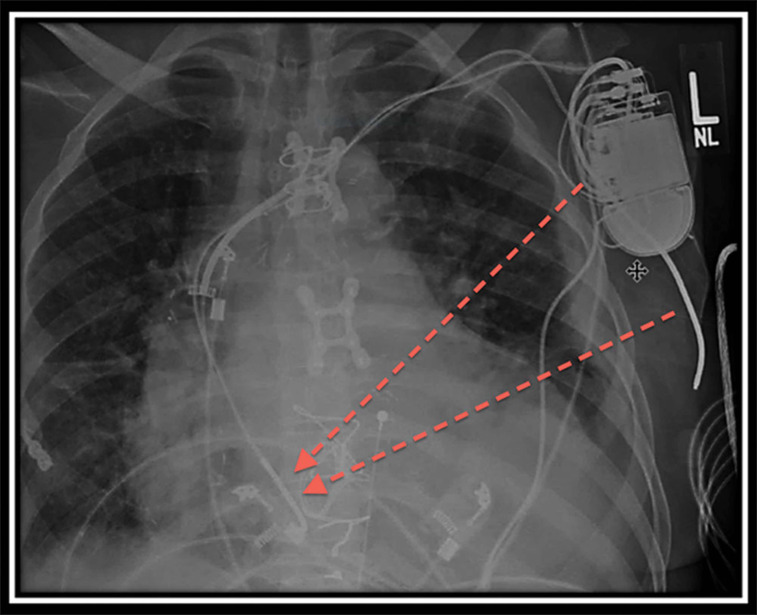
Anteroposterior chest X-ray depicting shock vector from the can/subcutaneous coil to the right atrial coil.
